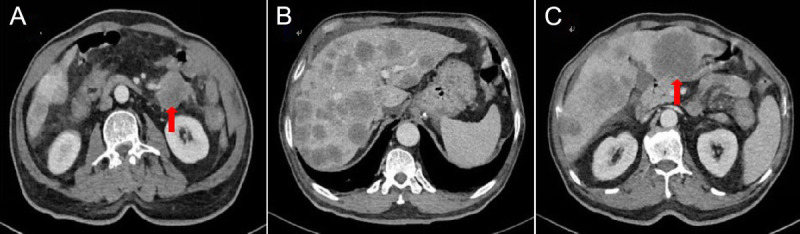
Contrast-enhanced abdominal CT revealed diffuse heterogeneous wall thickening of the left-sided jejunal bowel loops with luminal narrowing (A), suspicious for malignant infiltration, along with multiple hypodense space-occupying lesions in the liver consistent with metastatic involvement (B). The lesion in the left lobe of the liver adjacent to the stomach is the target of this EUS-FNA procedure (C).
